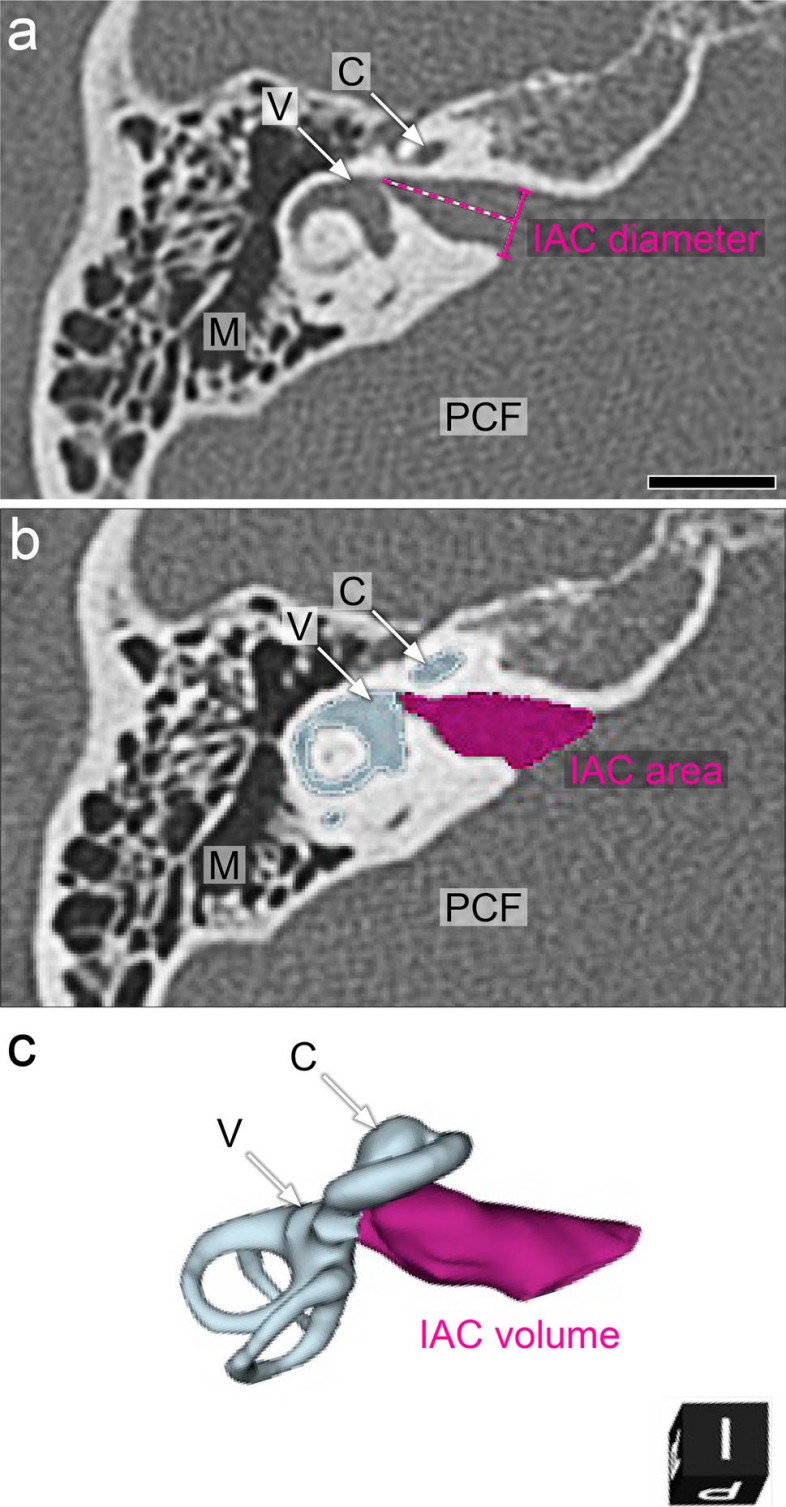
Exemplary measurements of the internal auditory canal (IAC) diameter and volume of a normal inner ear in an axial section of a high-resolution computed tomography. A At the *porus acusticus internus*, the IAC diameter (magenta line) was determined perpendicular to the longitudinal axis (dashed magenta line) of the IAC. Scale bar: 10 mm. B Exemplary segmentation of the IAC (magenta area). The cochlea (C) and vestibule (V) are outlined in light blue. C 3D reconstruction of the IAC (magenta) and the adjacent inner ear (cochlea, vestibule; light blue) M, mastoid. PCF, posterior cranial fossa
